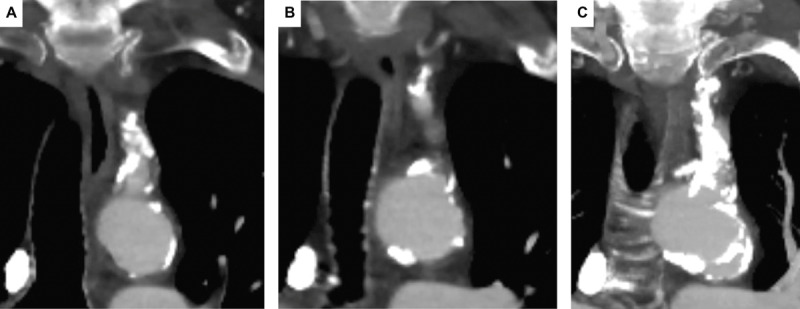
Computed tomography angiogram of the chest with visualization of the aortic arch and its branches, specifically (A) the proximal aspect of left subclavian artery with calcific stenosis, (B) the mid portion of left subclavian artery close to origin of vertebral artery, and (C) maximal intensity projection image showing distribution of calcification in the left subclavian artery.
